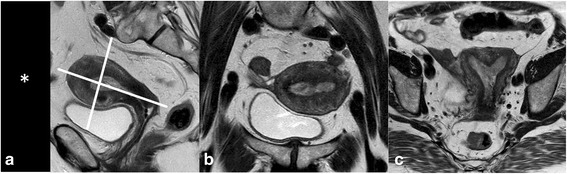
Basic imaging technique in stage Ia endometroid cancer. Anterior sat bands (*) are used to reduce artifacts from breathing. Sagittal T2WI (a) render an overview of the pelvis including the distal lumbar region. The image displaying the distended uterine cavity is used as reference for sections perpendicular (b) and along the long uterine axis (c). DWI are used in same plane as in b. The tumour displays intermediate SI on T2WI and shows restricted diffusion
